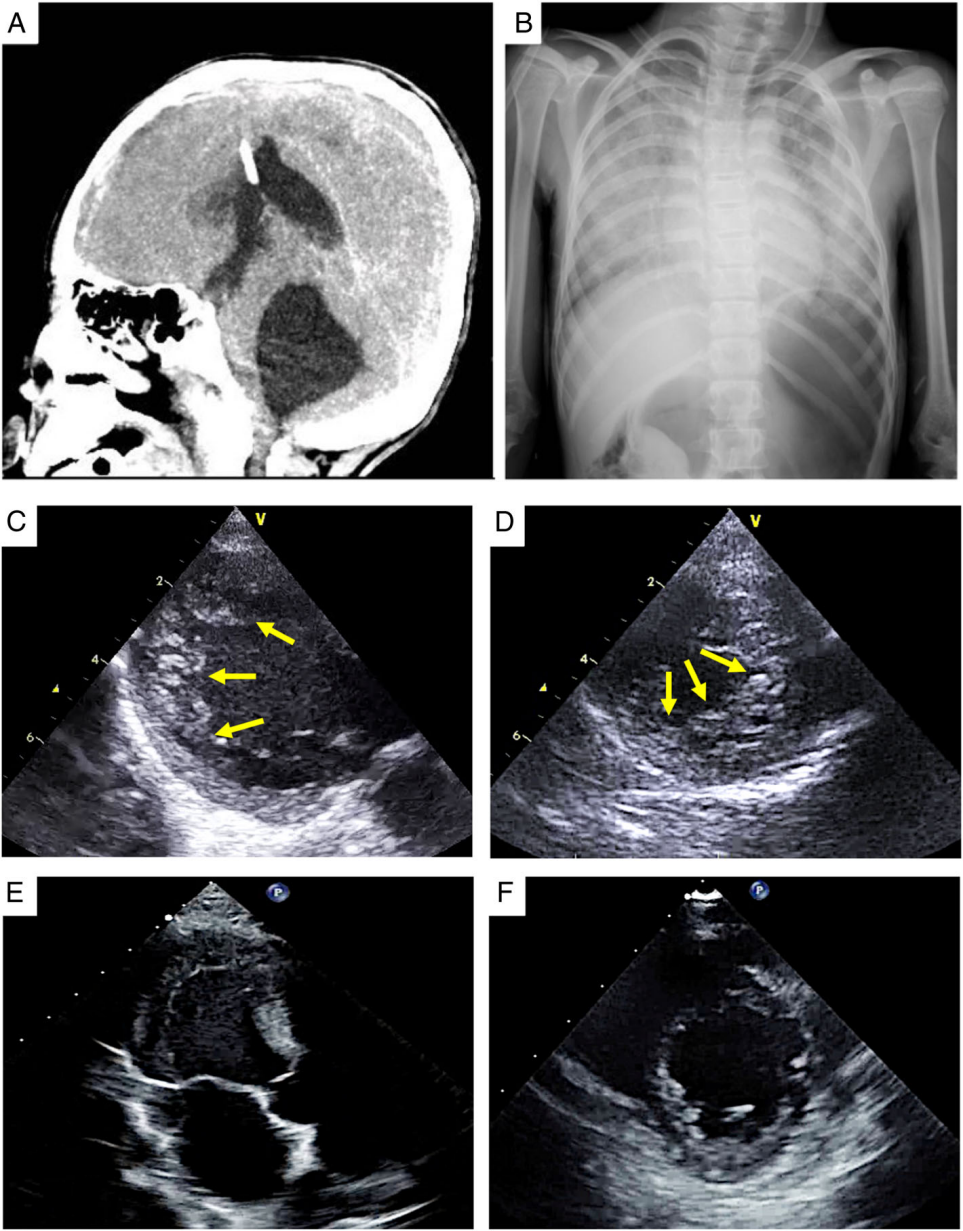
Head CT image (a), chest radiograph (b), and TTE images showing prominent trabeculations in the LV (c and d), subsequently, trabeculation no longer observable (e and f). TTE depicts heavy prominent trabeculae in the LV, predominantly in the apex, in the parasternal long axis view (c) and short axis view (d) on the 3rd day of illness. After 3 months, prominent trabeculation of the LV was no longer observable in the parasternal long axis view (e) and short axis view (f). The arrow indicates prominent trabeculations
